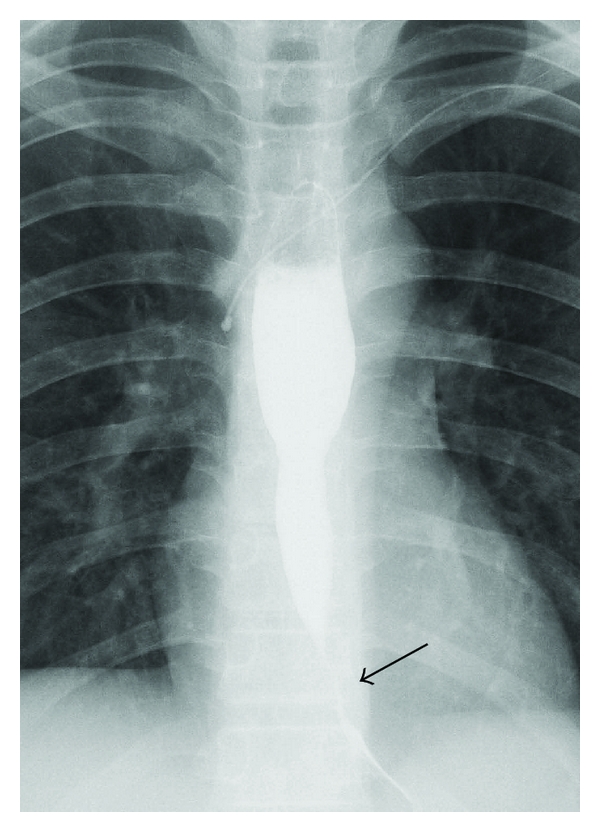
Chest radiograph obtained in the barium-swallowing examination showing stricture of the distal oesophagus. The black arrow indicates the oesophageal stenosis.
